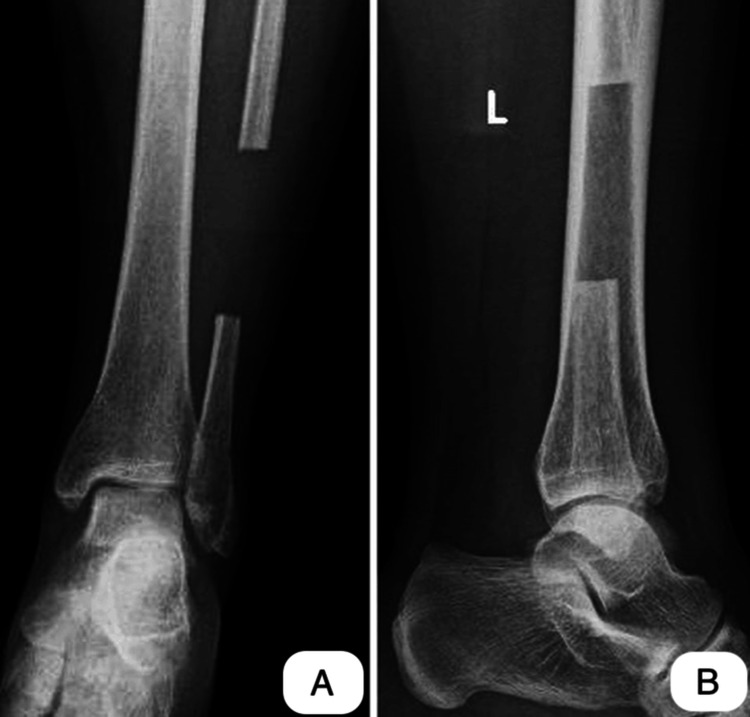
X-ray view after surgery A - antero-posterior view B - lateral view
